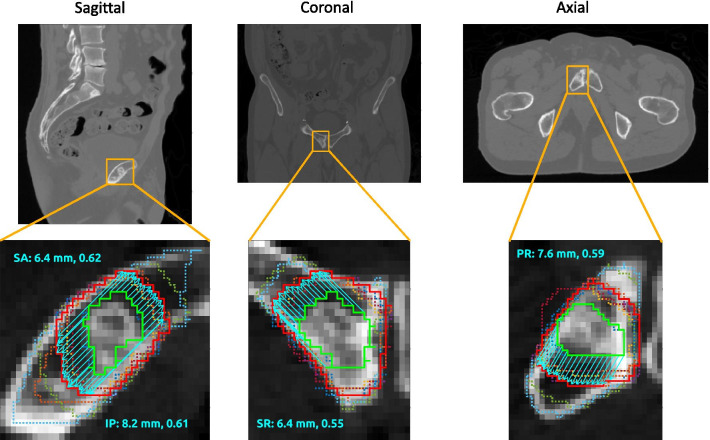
Consensus margin and margin deviation for a metastasis in the pubic symphysis without an extraosseous component: CT and overlaid contours for a patient with a metastasis in the pubic symphysis. The GTV and STAPLE CTV are represented by the solid green and red contours, respectively; the expert contours are dotted. The directions of potential intraosseous extension, indicated by the cyan vectors, are termed *superior-anterior* (SA), *inferior-posterior* (IP), *superior-right* (SR), and *posterior-right* (PR). The text annotations report the consensus margin (mm) followed by the margin deviation (dimensionless) for each direction
